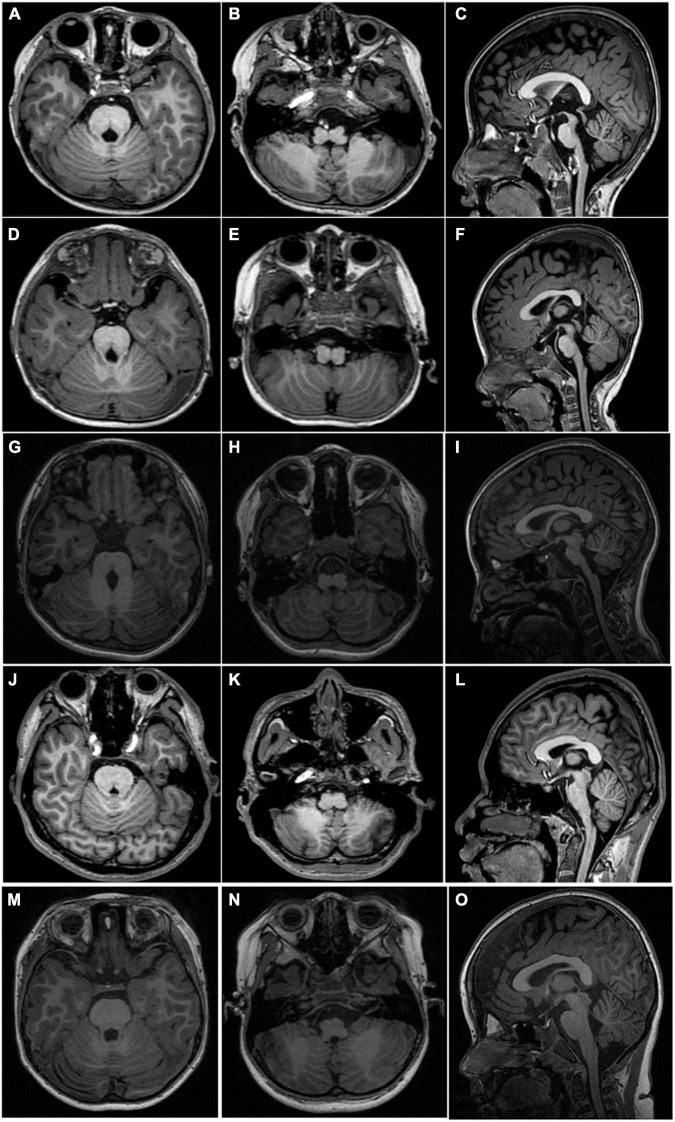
MRI scan acquired from four patients and a normal 6-year-old control. Axial T1WI shows a deep posteromedian cleft of the pons and a butterfly-like morphology of the medulla oblongata (left two columns, first four rows) in all patients. Sagittal T1WI shows flat pons and medulla oblongata and an enlarged fourth ventricle (C,F,I,L) in all patients. The upper panel, (A–C), taken from P1; middle panel, (D–F), taken from P2; (G–I) taken from P5; the lower panel, (J–L), taken from P6. Axial T1 (M,N) and sagittal T1 (O) weighted images show normal-shaped pons and medulla of a normal 6-year-old boy.
